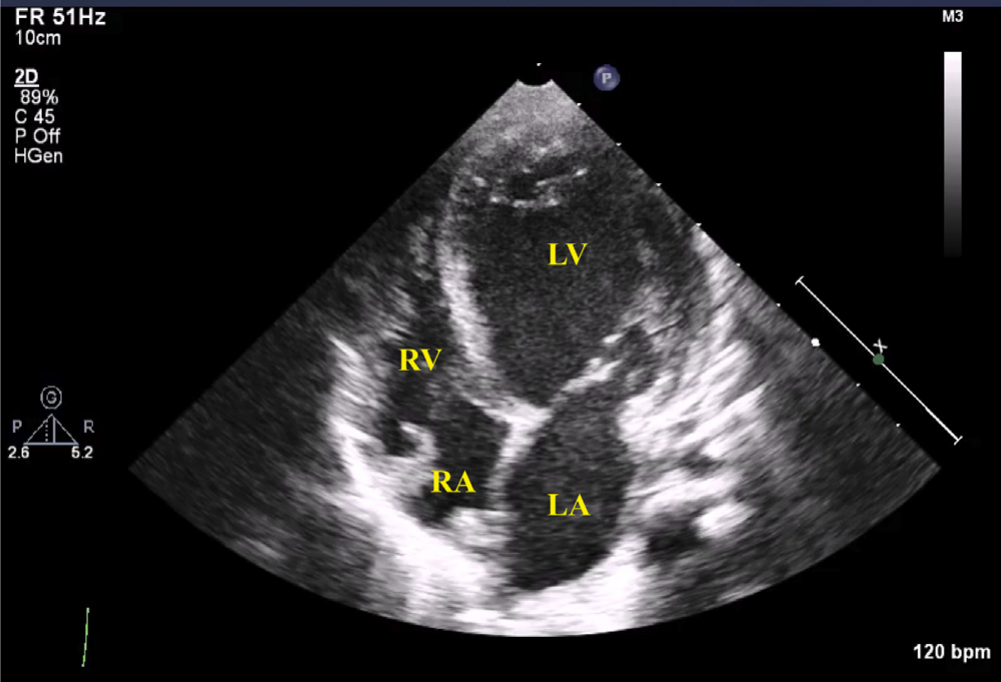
Transthoracic echocardiography from the apical 4-chamber window showing dilated left atrium and left ventricle. *LA*, Left atrium; *LV*, left ventricle; *RA*, right atrium; *RV*, right ventricle.
